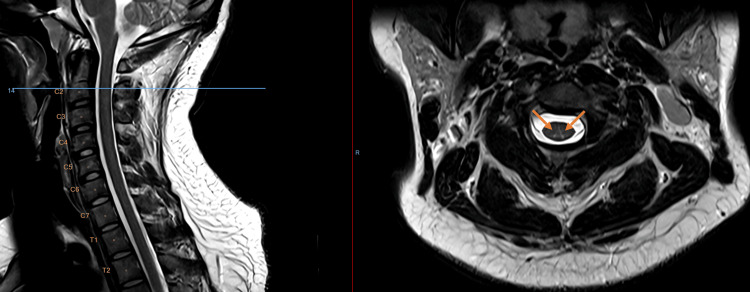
Axial T2-weighted MRI (3T) imaging of the cervical spine (C1-2) Linear T2 hyperintensities of the dorsal columns of the cervical spine forming an “inverted V sign” (orange arrows), characteristic of functional vitamin B₁₂ deficiency caused by N₂O toxicity, extending from C1 to C5. The hyperintensities are not visible on the sagittal plane because of spatial resolution.
